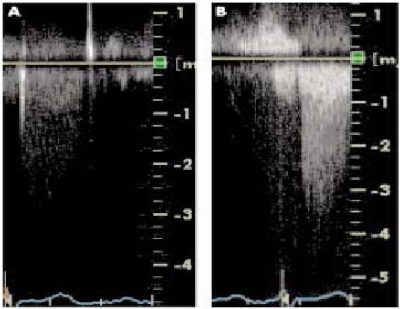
Aortic stenosis flow Doppler tracing (A) without and (B) with contrast enhancement.
